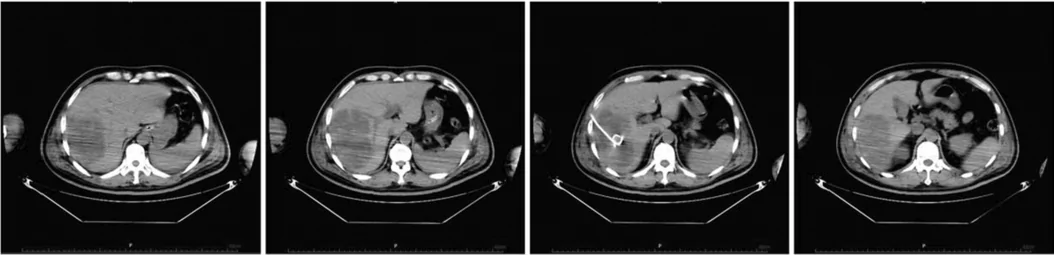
The abdominal CT of the patient on the eighth day of admission showed a slightly low‐density image of the right lobe of liver, about 113 × 73 mm in size. And the liver puncture catheter placement for drainage.
